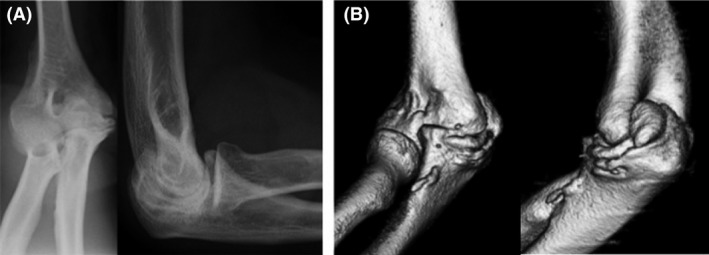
Bony protuberances are seen on the anteroposterior and lateral radiographs (A) and 3D CT (B).
